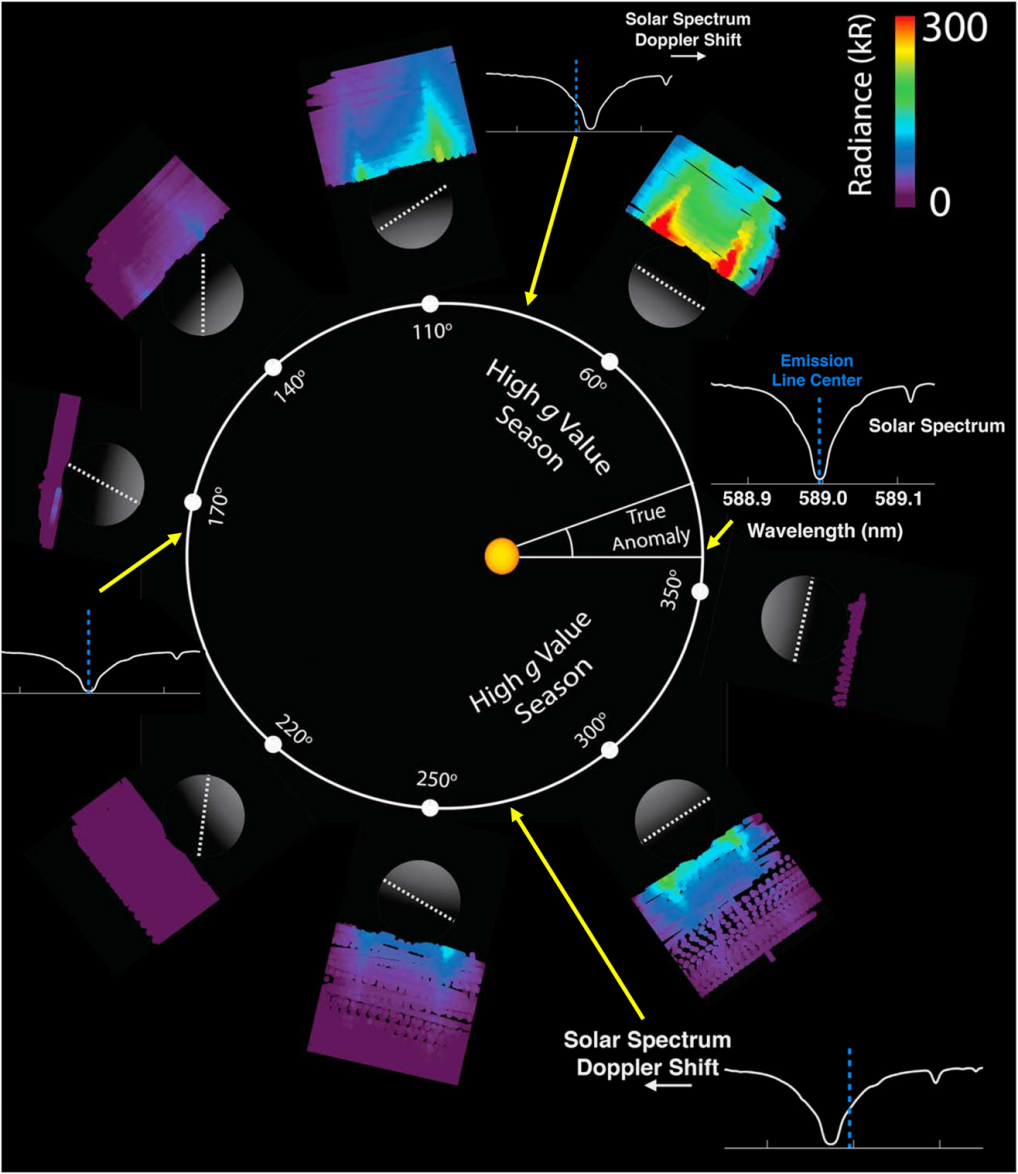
The observed UVVS neutral sodium emission projected onto Mercury's orbital plane, showing the sodium tail which forms during the high *g*‐value portions of Mercury's eccentric orbit. The doppler shift of the solar spectrum at the D2 sodium resonance line (dotted blue line) is also shown at TAAs of 0°, 90°, 180°, and 270°. The white dotted line on Mercury, shows the location of the cold‐pole longitudes (longitudes with lower than average temperatures, Peplowski et al., 2014). Adapted from Cassidy et al. (2015, 2016). UVVS, UltraViolet and Visible Spectrometer.
